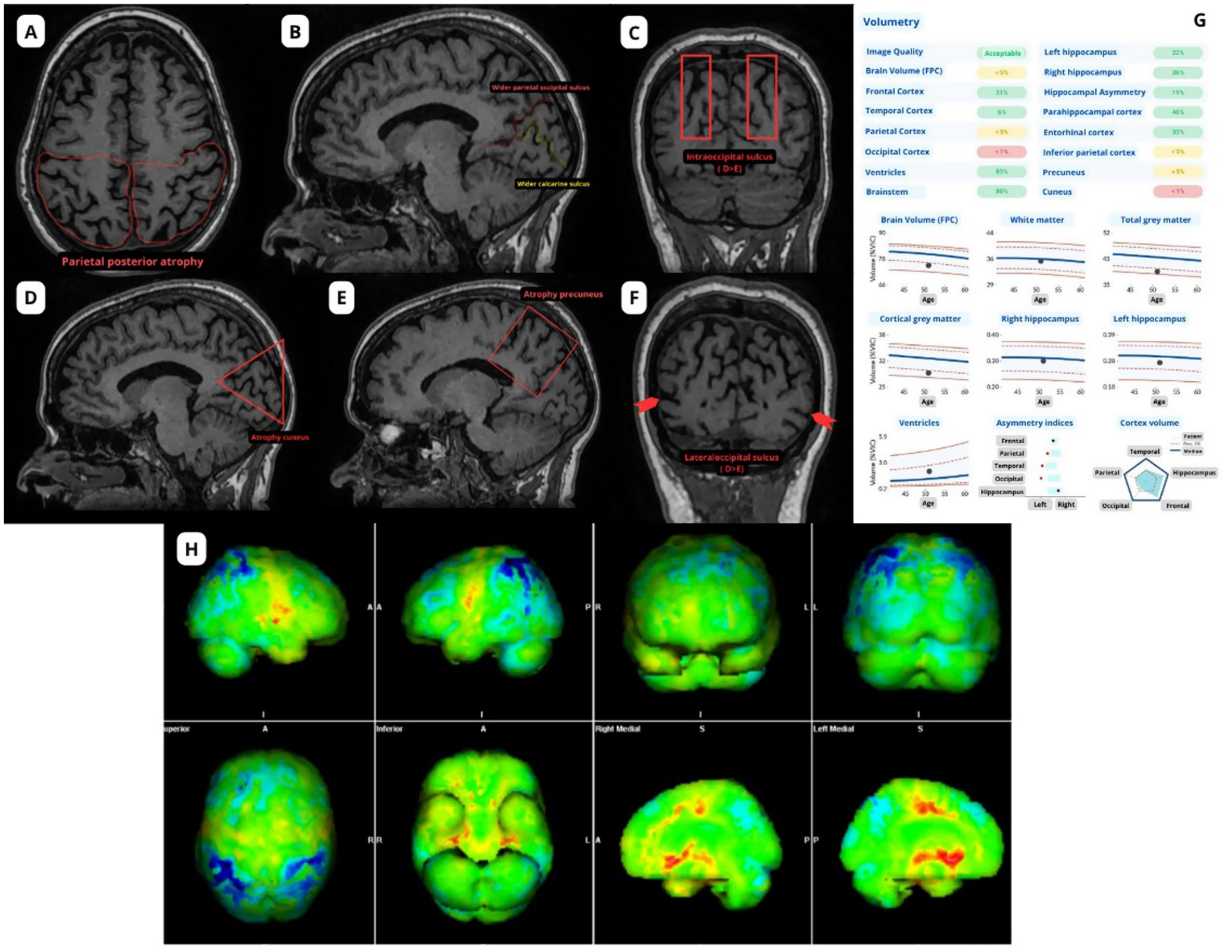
Main anatomical changes in posterior cortical atrophy. Images were acquired using a GE scanner with a magnetic field strength of 1.5 Tesla. A pre-contrast volumetric T1-weighted sequence was used to highlight the alterations from (A) to (F), as well as for volumetric analysis. (A) Atrophy of the posterior parietal region; (B) Enlarged parieto-occipital sulcus and calcarine sulcus; (C) Right intra-occipital sulcus wider than the left; (D) Cuneus atrophy; (E) Precuneus atrophy; (F) Right lateral occipital sulcus wider than the left; (G) Volumetry; (H) FDG PET: Bilateral posterior parietal hypometabolism in a case of mild cognitive impairment due to synucleinopathy, with visuospatial deficits.
